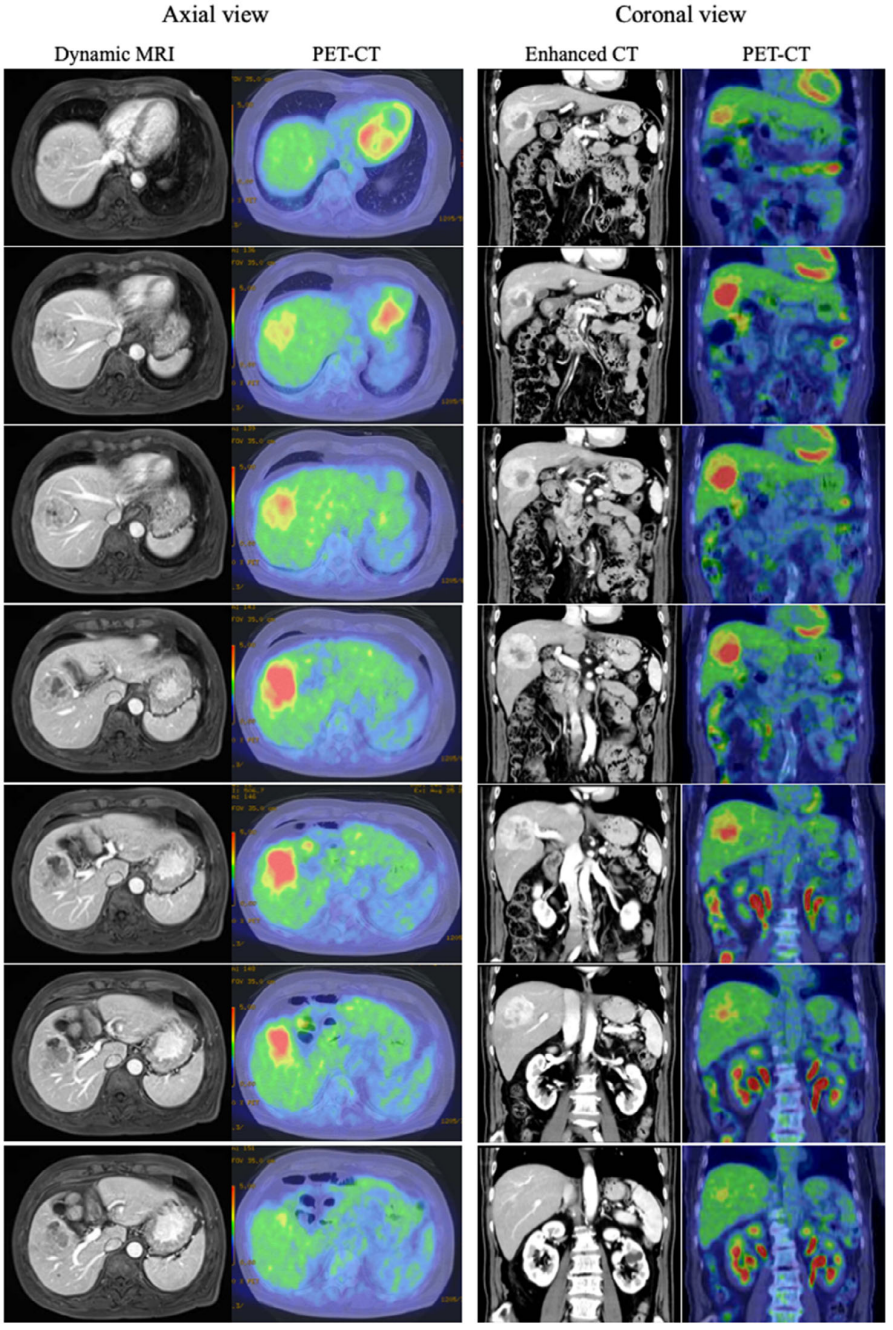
Comparison of findings of PET‐CT with dynamic MRI and enhanced CT. FDG‐PET/CT revealed a strong uptake in the corresponding NEC area in the tumor detected from dynamic MRI and enhanced CT, respectively.
